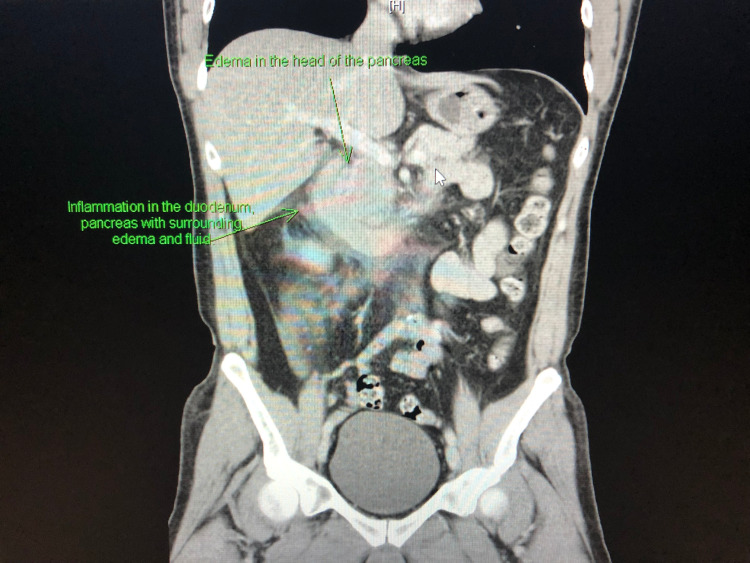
CT of the abdomen in a coronal view Computed tomography (CT) of the abdomen displaying edema at the head of the pancreas. There is also inflammation in the duodenum and pancreas with surrounding fluid and edema.
